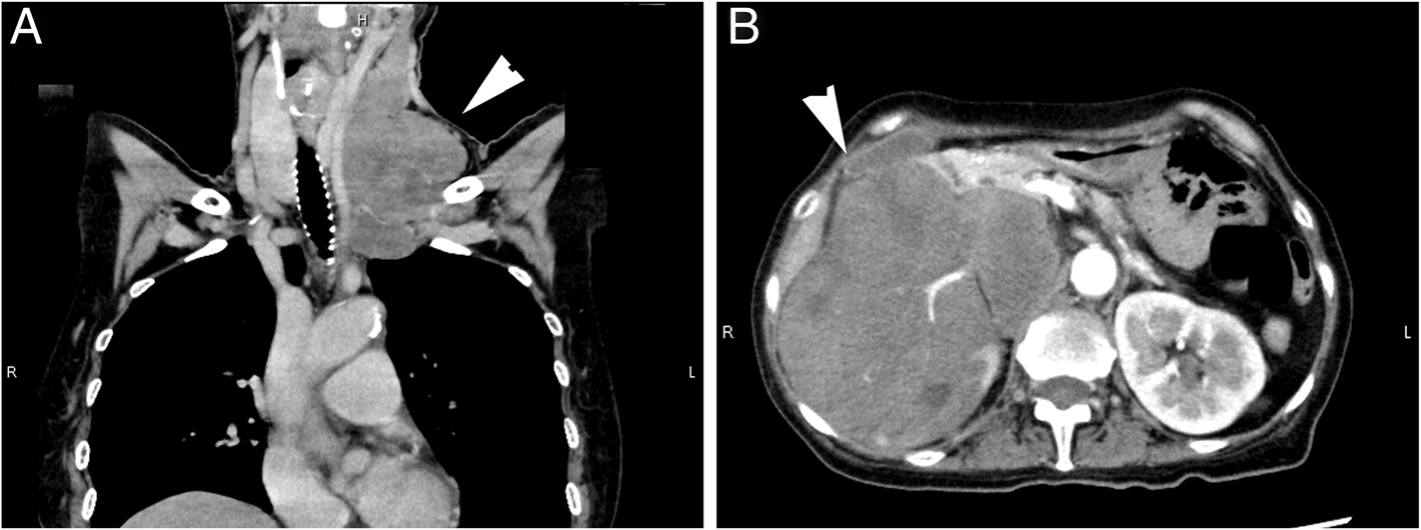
Clinical images: Computed tomography (CT), obtained before the biopsy, showing the swollen lymph nodes in the left neck (a, *white arrow head*) and a huge abdominal mass occupying the right kidney (b, *white arrow head*) as low-density masses
